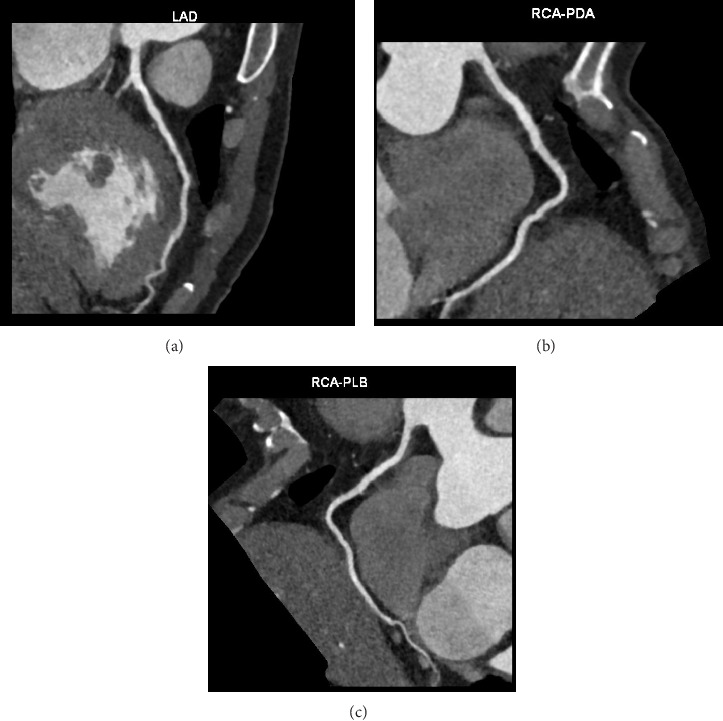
CT cardiac coronary angiography shows no significant coronary artery disease with a calcium score of zero. From left to right: (a) image shows left anterior descending (LAD) artery, (b) image shows right coronary artery-posterior descending artery (RCA-PDA),and (c) image shows right coronary artery-posterolateral branch (RCA-PLB).
